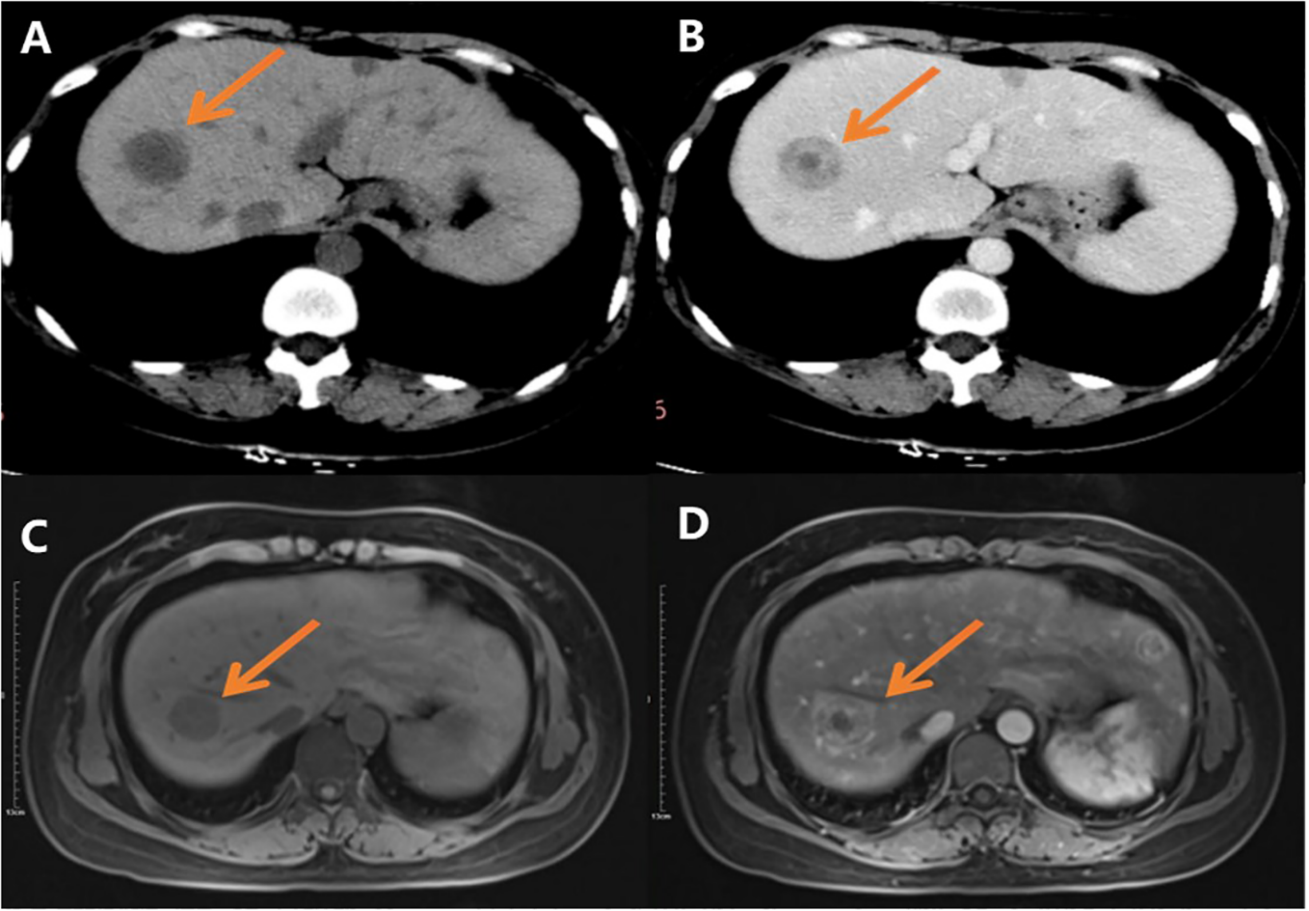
CT and MR images: (A) CT scan shows multiple round low-density shadows in the liver; (B) CT scan shows there is ring-shaped enhancement, and the “bull’s eye sign” can be seen after contrast administration; (C) Slightly low signal shadows in T1WI; (D) Slightly high signal shadows in T2WI.
